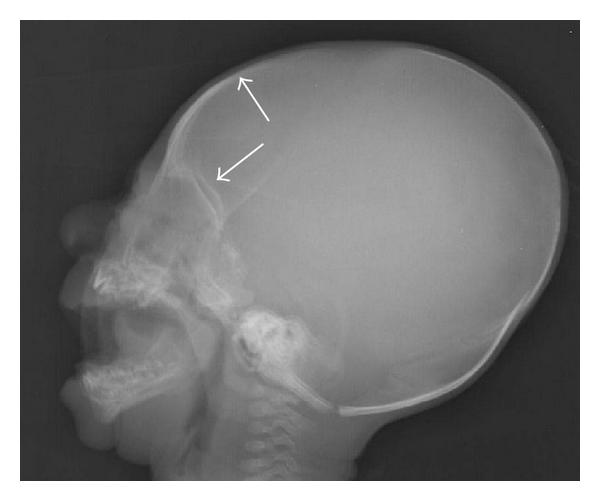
X-ray skull showing evidence of craniosynostosis (arrows) of frontal and coronal sutures.
